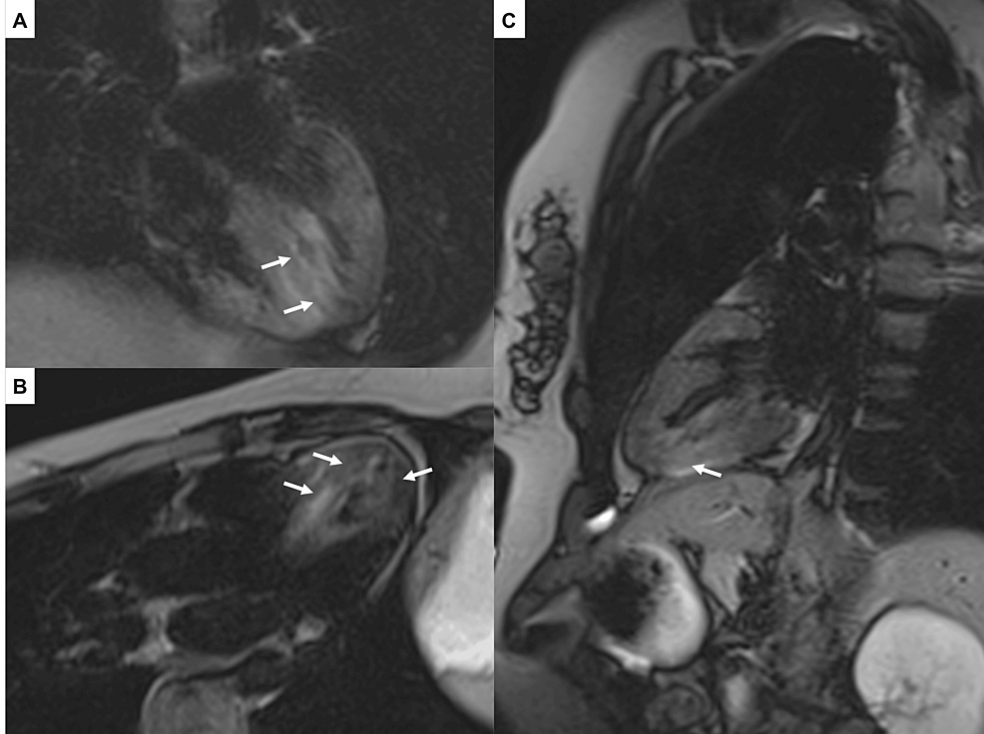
Cardiac magnetic resonance imaging Four-chamber (A), three-chamber (B), and two-chamber (C) view T2-weighted images. The white arrows indicate myocardial edema in mid-septal and apical septal, inferior, and lateral segments.
